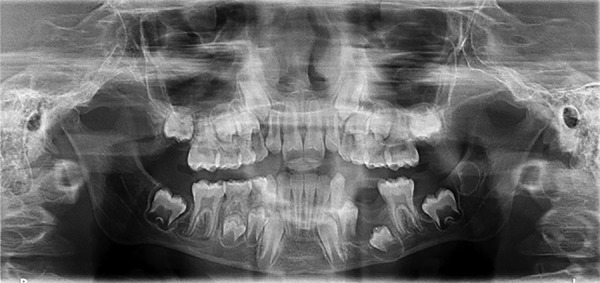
Orthopantomography at 3-month follow-up
